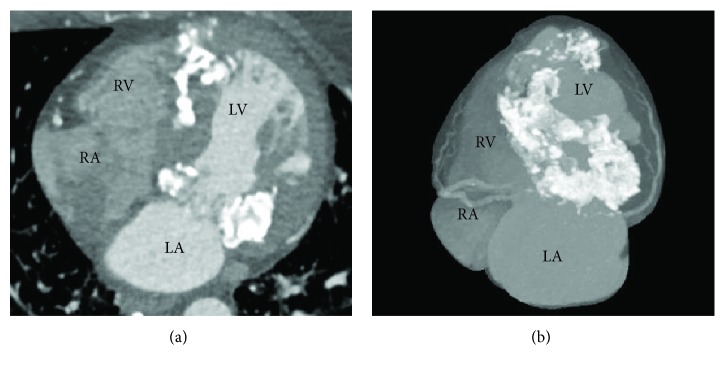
Computed tomography ((a) multiplanar reformatted (MPR) and (b) maximal intensity projection (MIP)) demonstrated extensive infiltrative calcification, circumferentially around the mitral annulus, extending posteriorly and septally into the myocardial apex. RA: right atrium; RV: right ventricle; LA: left atrium; LV: left ventricle.
